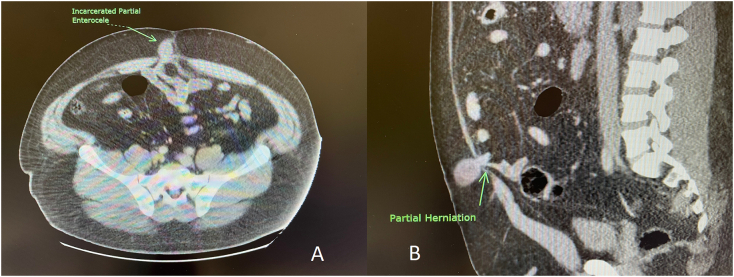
A and B: Computed Tomography scans of the abdomen (axial and sagittal cuts) demonstrating a hernia.
